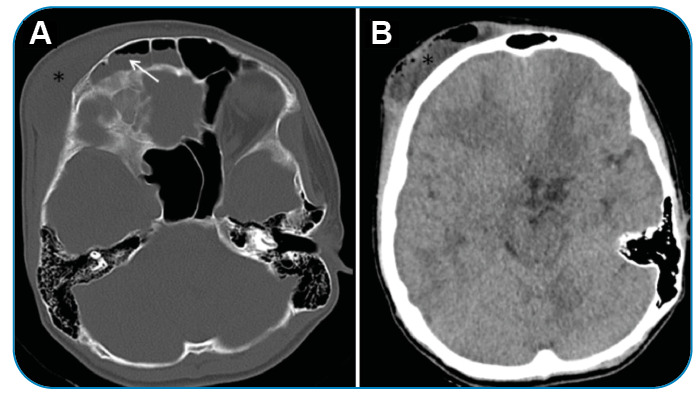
On CT images in the axial section, bone (A), and parenchyma (B) window, a lesion (asterix) consistent with a subperiosteal abscess is seen, containing air densities in the right frontal region, and density increases (arrow) in the right frontal sinus.
